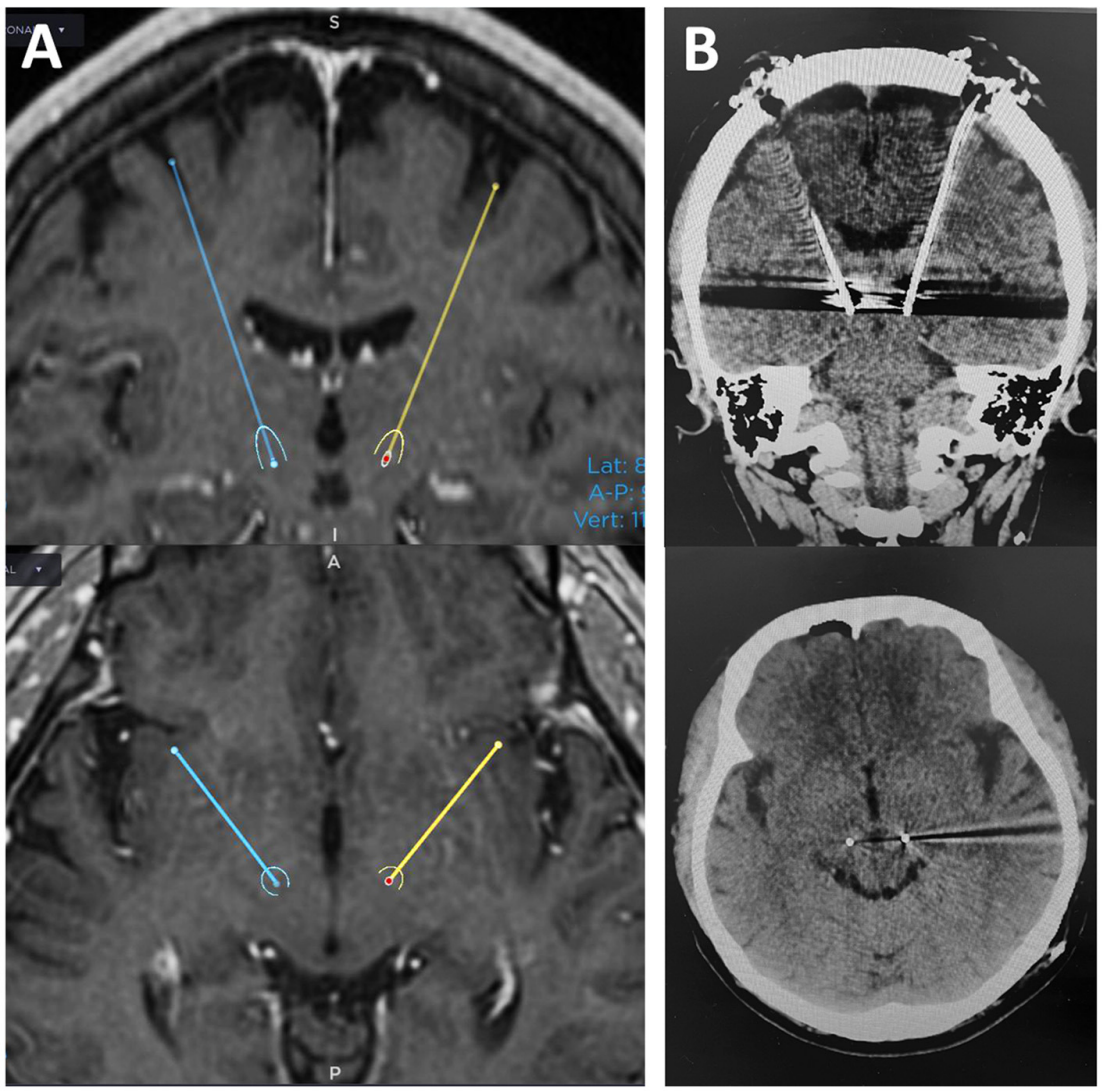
Preoperative planning and postoperative CT images in case 5. In this case, the target point (the ventral border of the STN) was 11 mm lateral to the midline, 2.5 mm posterior to the midpoint of the AC-PC, and 5 mm below the AC-PC line on both sides (A). Postoperative CT images show the lead was placed almost as planned (B).
